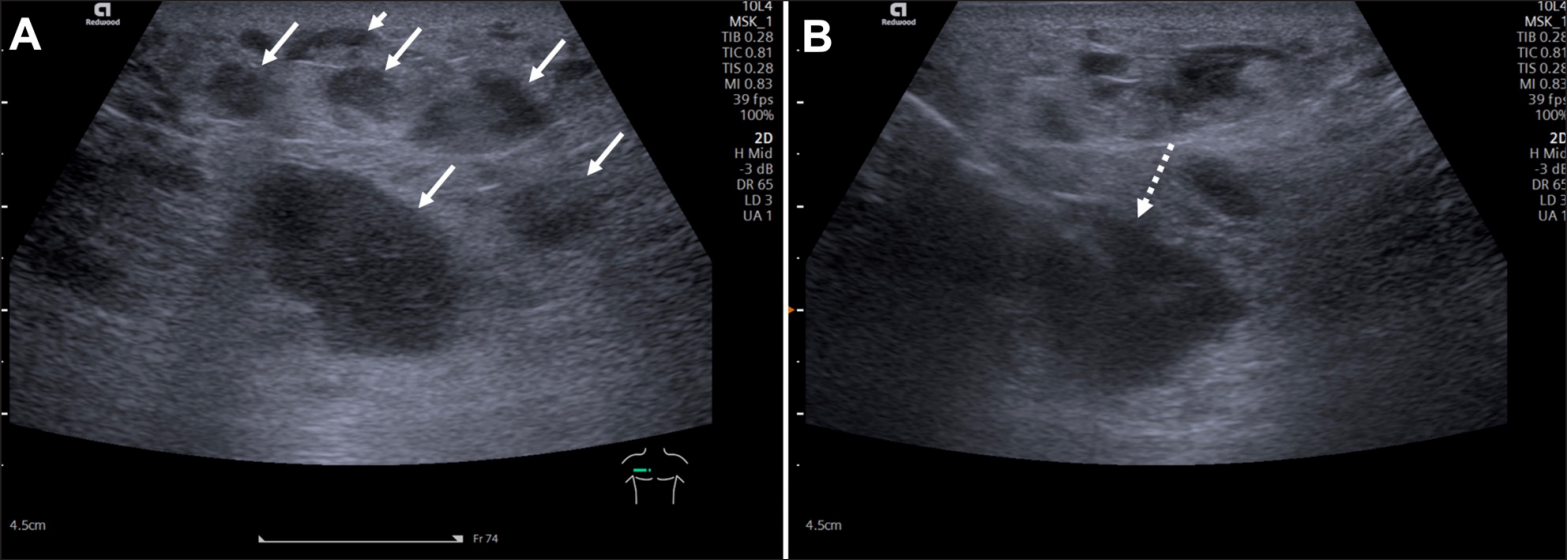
The patient underwent chemotherapy with R-CHOP, after which he was clinically well, with complete resolution of lesions on follow-up CT.
